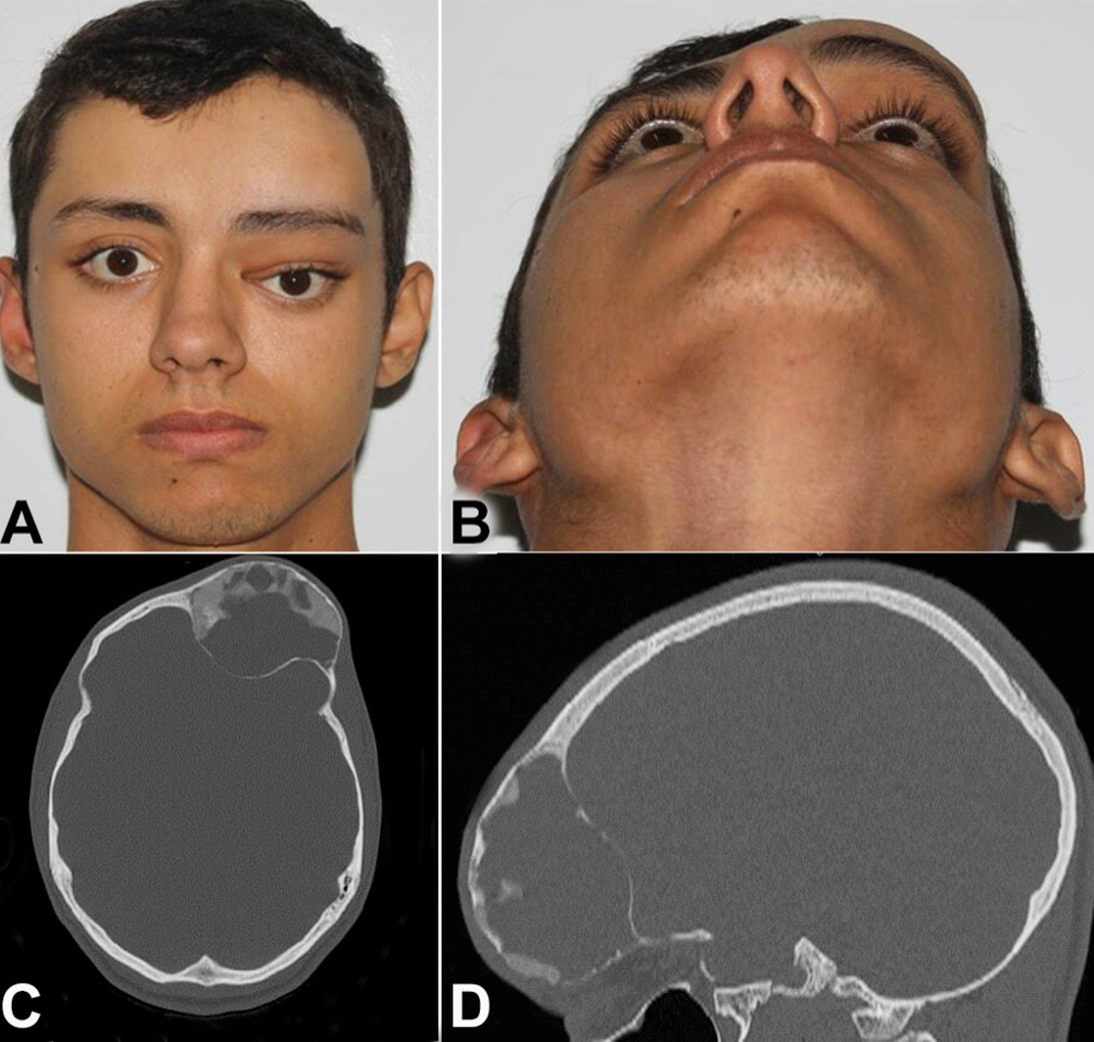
Clinical and imaging aspects. A – front view showing ocular dystopia; B – infero-superior view, highlighting the expansive aspect of the lesion; C – CT axial section, showing a mixed unilocular lesion; D – CT sagittal section, showing the preservation of the anterior and posterior cortices.
